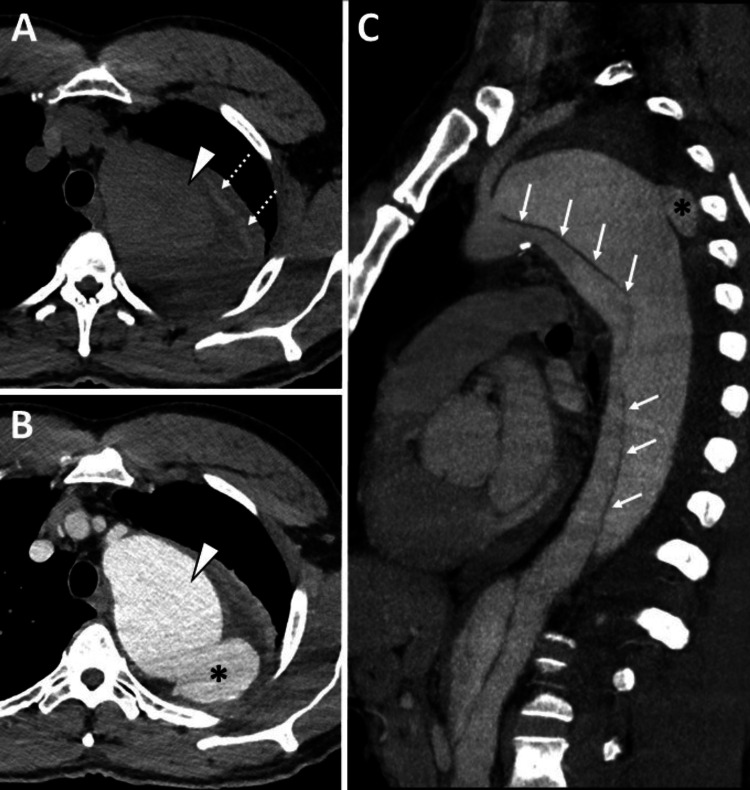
CT angiography of the chest was performed after the administration of 80 mL of intravenous contrast iopamidol 76% (Isovue 370, Bracco) in the right upper extremity. (A) Unenhanced axial CT, (B) contrast-enhanced axial CT, and (C) oblique aorta MIP (maximum intensity projection) demonstrate a type B aortic dissection with the dissection flap extending distally from the origin of the left subclavian artery (solid white arrows in C). Additionally, there is an intramural hematoma along the proximal descending thoracic aorta (dashed white arrow in A) and a localized saccular outpouching indicating contained rupture (black asterisk in B and C) from the posterior false lumen of the proximal descending thoracic aortic dissection with aneurysmal dilation (white arrowhead in B).
